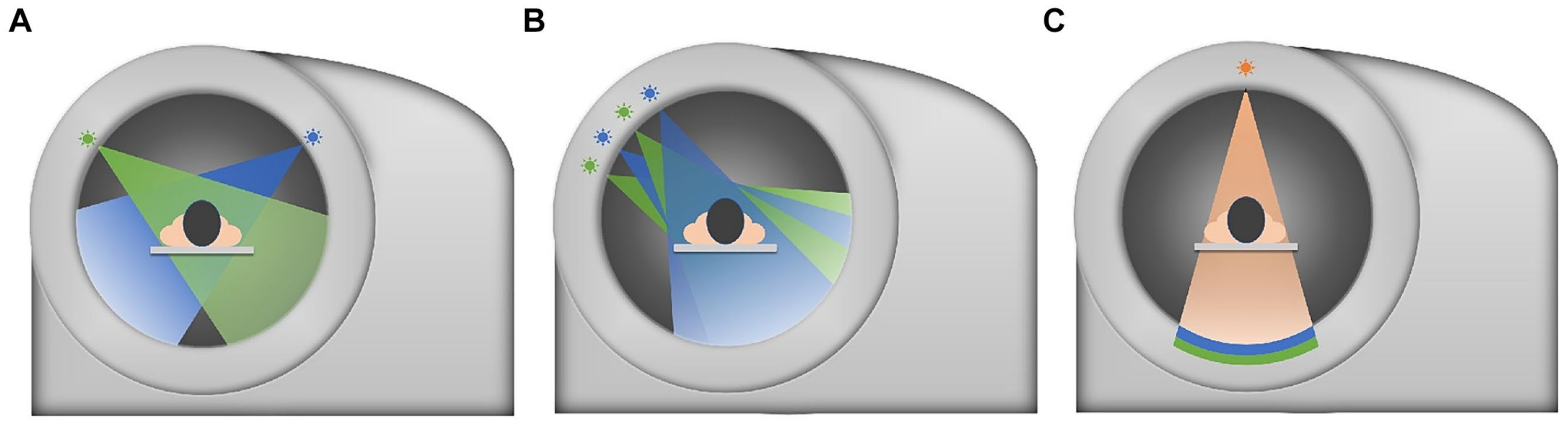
Sketch map of three main types DECT. (A) Dualsource-DECT: Composed of two independent x-ray tubes and two independent detectors. (B) Singlesource-DECT: Containing a dedicated generator that can quickly switch between low-energy and high-energy projections that are collected separately by a fast sampling-detector. (C) Duallayer-detector DECT: Composed of a two-layers-detector with different energy sensitivity.
